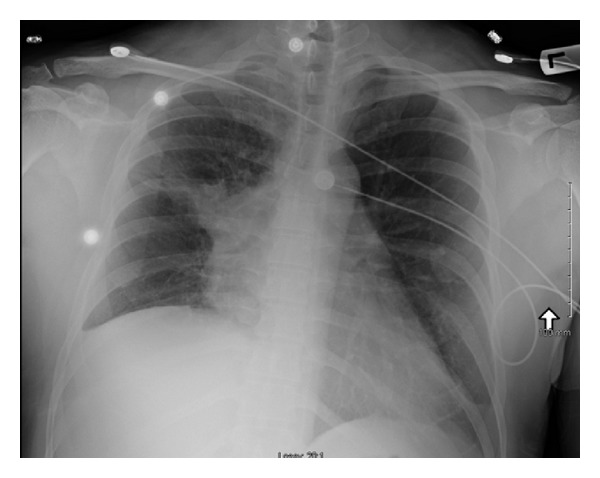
Chest X-ray showing a large right hilar mass extending to the right upper lobe with associated fine loss in the right lung. There is a right pleural effusion seen as well.
